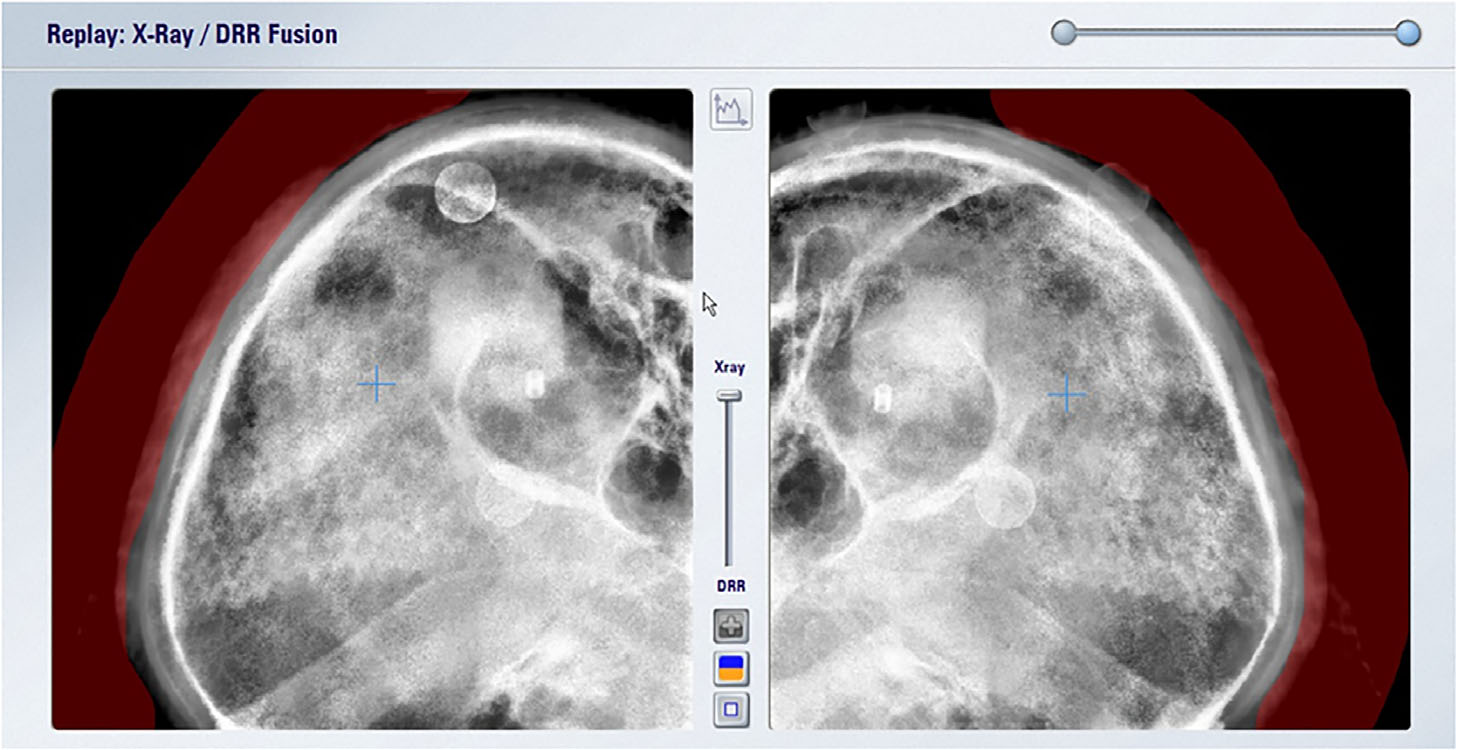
Masking process when the parietal area is included in the ExacTrac X‐ray system. The masking area is red Abbreviation: DRR, digitally reconstructed radiograph.
